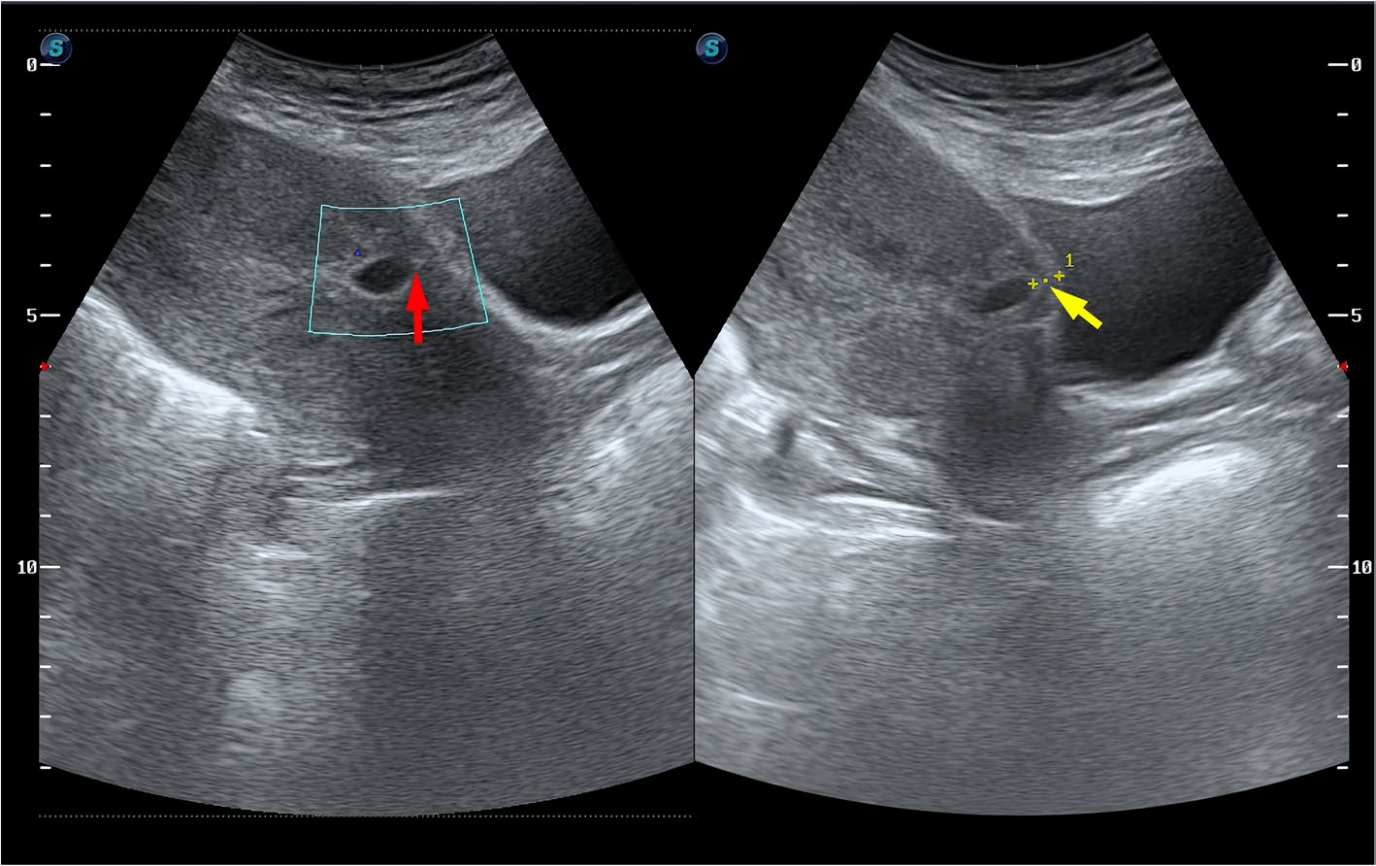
Ultrasound image of CSP. Ultrasound shows that the gestational sac located in the anterior lower uterine segment (red arrow) and there was a lack of normal myometrium between the gestational sac and the bladder (yellow arrow)
